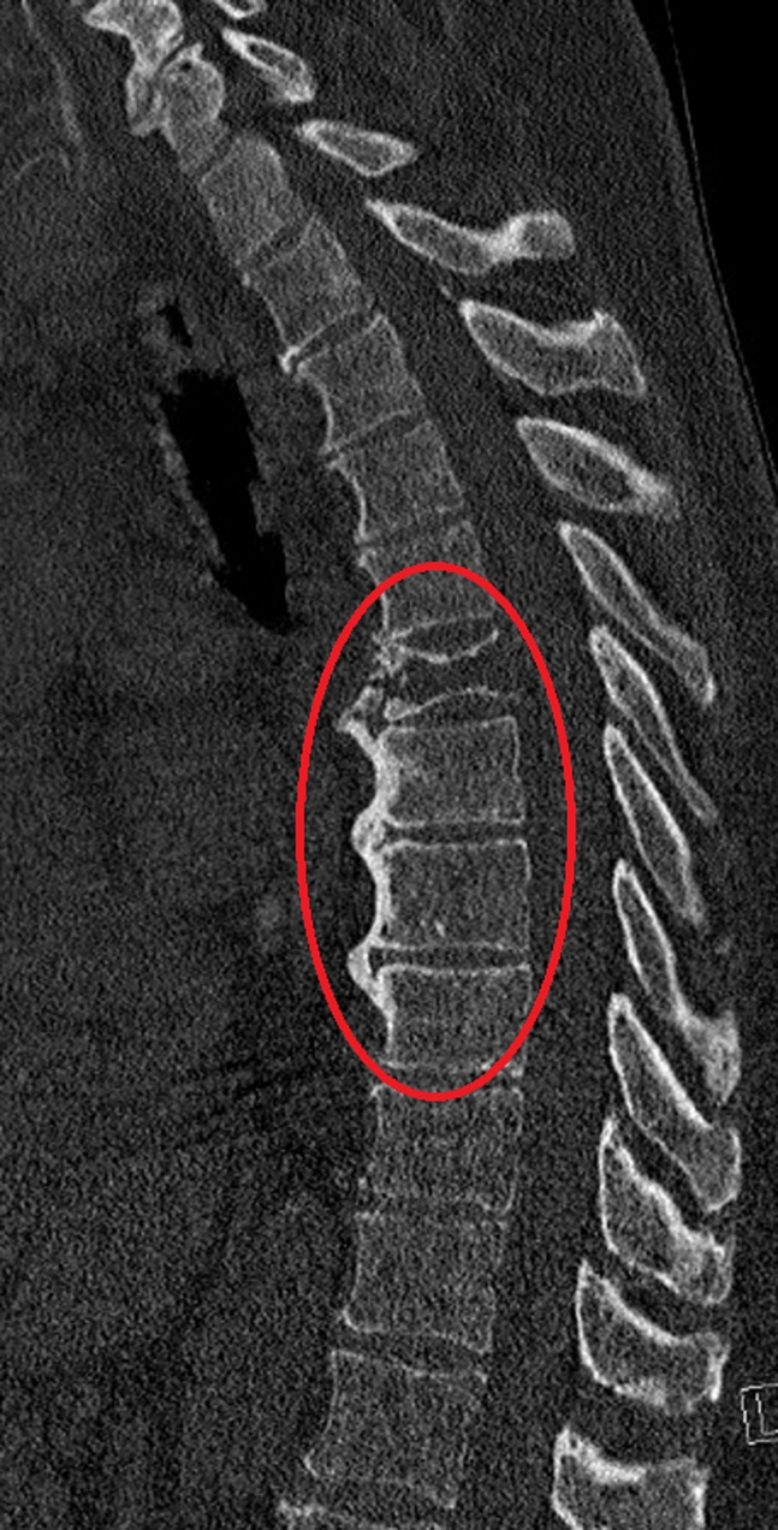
Computed tomography scan without contrast of thoracic spine A computed tomography scan without contrast of thoracic spine showing multiple myelomatous involvements of the T6 and T7 vertebra including compression deformity and ventral epidural extension at the T6 level
